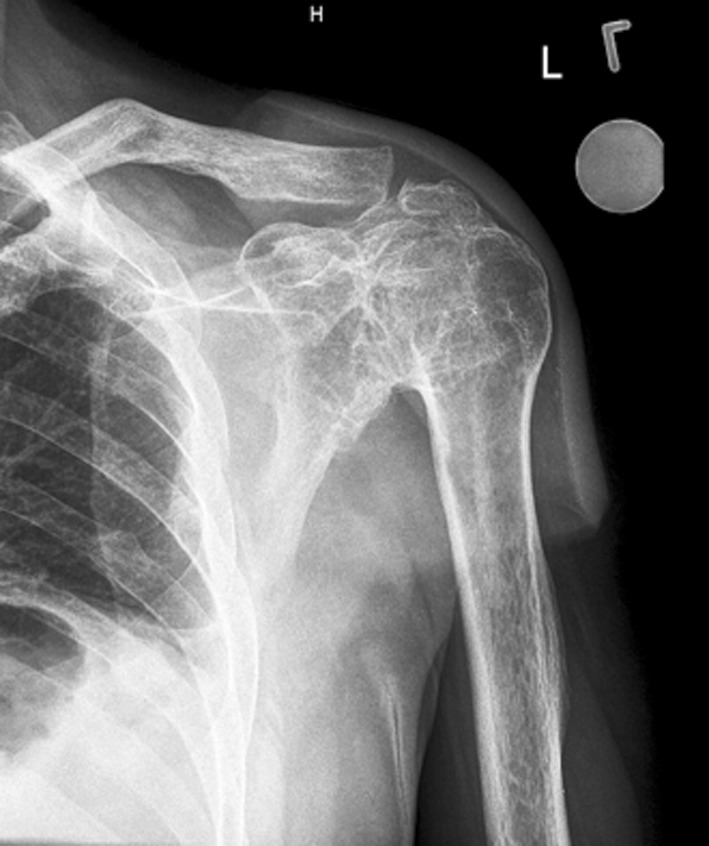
X-ray of the left shoulder of patient 1, 18-years after shoulder arthrodesis
